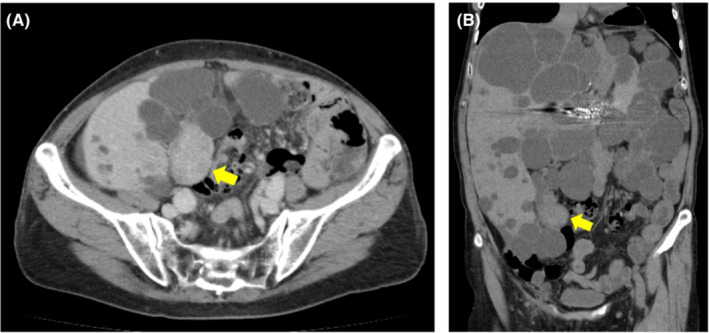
(A, B) Abdominal computed tomography scan. The yellow arrow reveals a 40‐mm‐large hyper dense mass inside a hypodense structure that is seemingly a cyst.
